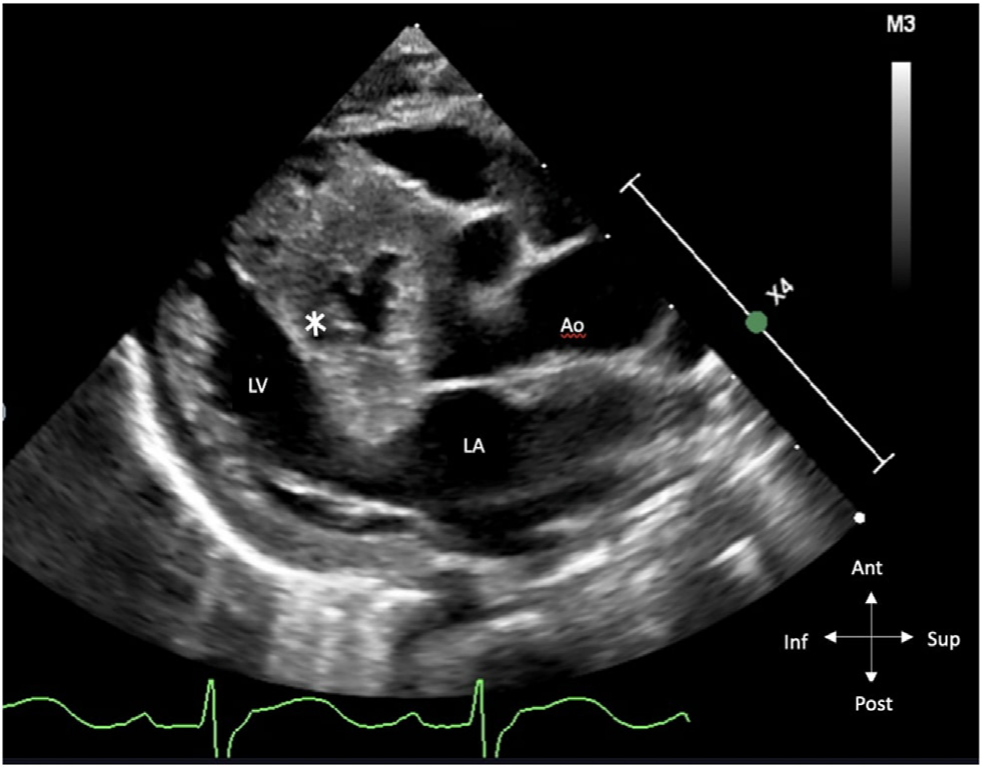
Postnatal two-dimensional transthoracic echocardiogram, parasternal long-axis view, systolic phase, demonstrates a severely hypertrophied and aneurysmal RV with the ventricular septum encroaching into the left ventricular outflow tract (*asterisk*). *Ao*, Aorta.
